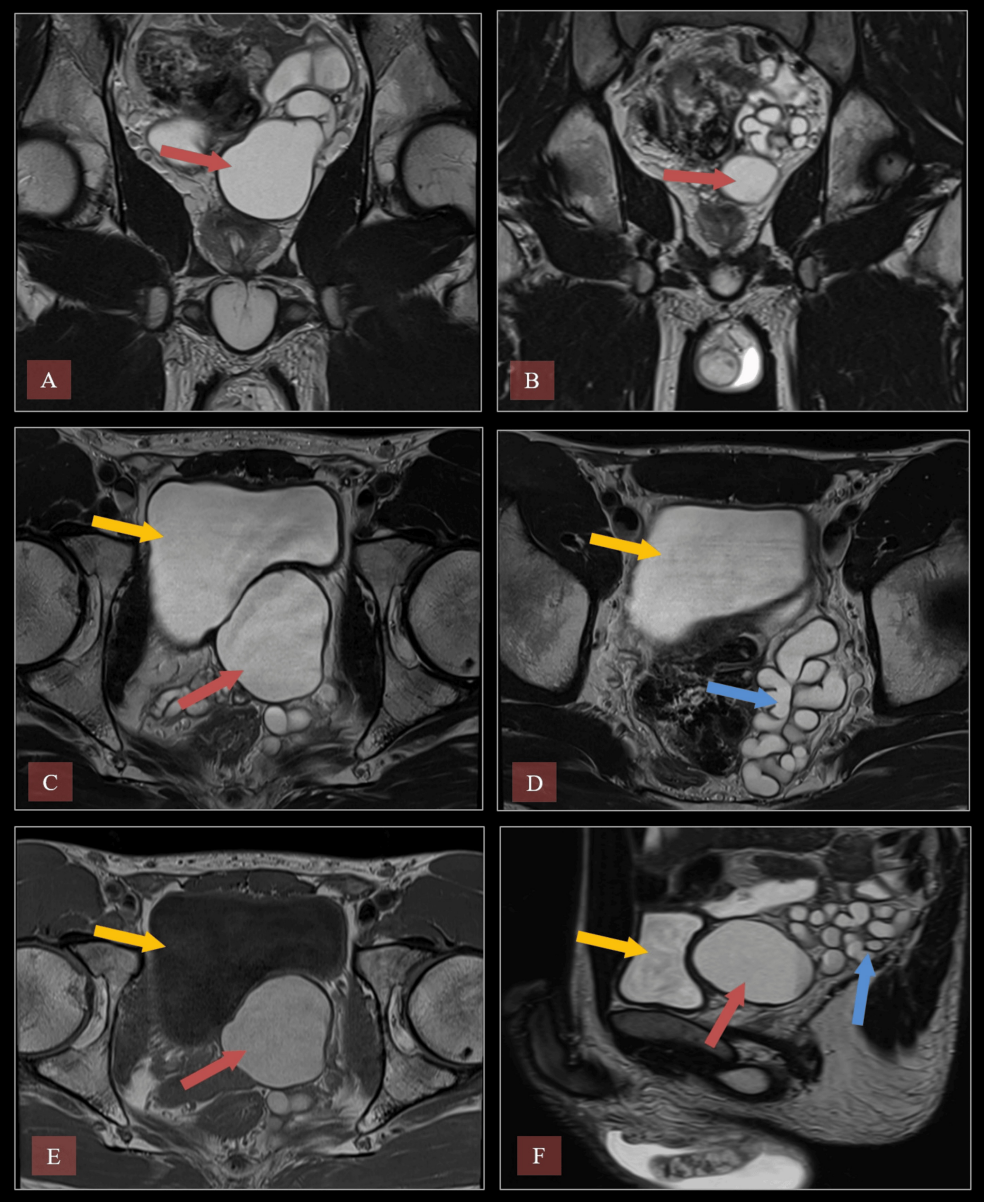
MRI of the pelvis demonstrating a left seminal vesicle cyst and dilated seminal vesicle. (A) Coronal T2-weighted MRI. The red arrow indicates the seminal vesicle cyst. (B) Coronal T2 TSE. The red arrow indicates the seminal vesicle cyst. (C) Axial T2 TSE. The yellow arrow indicates the bladder, and the red arrow indicates the seminal vesicle cyst. (D) Axial T2 TSE. The yellow arrow indicates the bladder, and the blue arrow indicates the dilated seminal vesicle. (E) Axial T1 TSE. The yellow arrow indicates the bladder, and the red arrow indicates the seminal vesicle cyst. (F) Sagittal T2 TSE. The yellow arrow indicates the bladder, the red arrow indicates the seminal vesicle cyst, and the blue arrow indicates the dilated seminal vesicle. TSE: turbo spin echo
